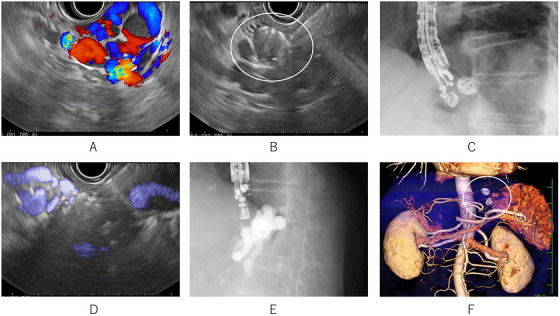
EUS‐guided coil deployment with sclerotherapy. A: Color Doppler EUS showed complex blood flow in the gastric varices. B: Coils were deployed and placed in the gastric varices under EUS guidance (area circled). C. Fluoroscopic image of the coil placement. D. Color Doppler showed that variceal blood flow was almost completely absent at the site where the coil was placed. E. After coil placement, a sclerosant (ethanolamine oleate) is injected to induce thrombosis of the remaining variceal lumen and blood supply pathway. F: Coils were placed at two sites, and the gastric varices completely disappeared (area circled).
